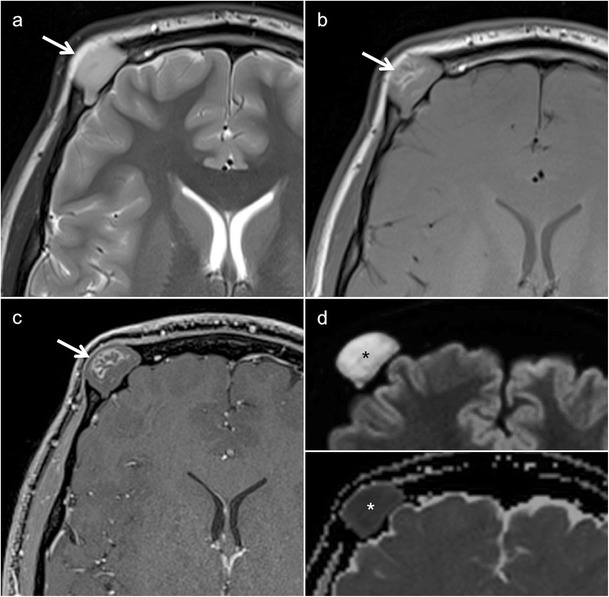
Intraosseous dermoid cyst (DC) in a 17-year-old male presenting as a gradually enlarging right supraorbital swelling since three years. a Cystic lesion in the right frontal bone (*arrow*) with non-lipomatous contents on axial T2. On T1 (b) and fat-saturated contrast-enhanced T1 (c), the lesion appears hypointense and displays intracystic serpiginous hyperintense areas which correspond to haemorrhage, high protein content or saponification (*arrows*). d DWI reveals high signal on the b = 1000 image (*asterisk*, *upper image part*) and low signal on the ADC map (*asterisk*, *lower image part*, ADC = 0.6 × 10^−3^ mm^2^/s), compatible with restricted diffusion and characteristic of an epidermoid cyst
